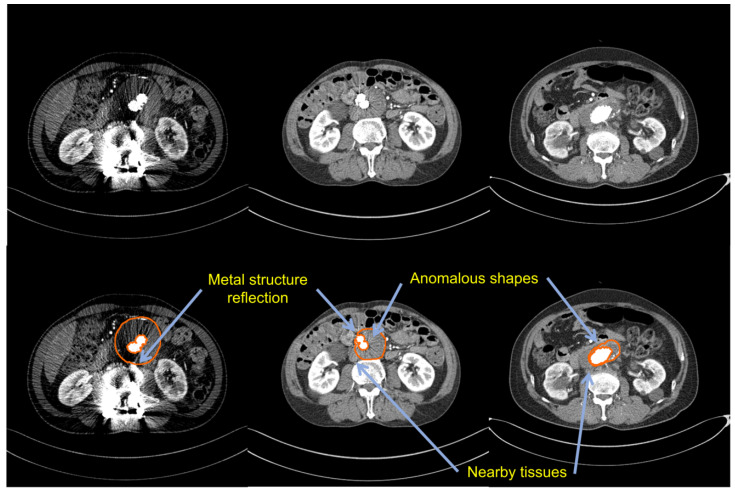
A sample training image from our dataset: the first row shows the original images; the second row describes the challenging features of CTA images.
